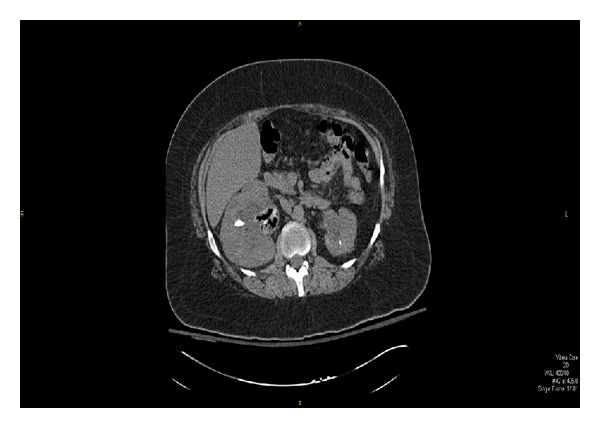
CT scan of the abdomen and pelvis showing marked right hydronephrosis with calculi, perinephric fat stranding, and gas in the renal pelvis.
